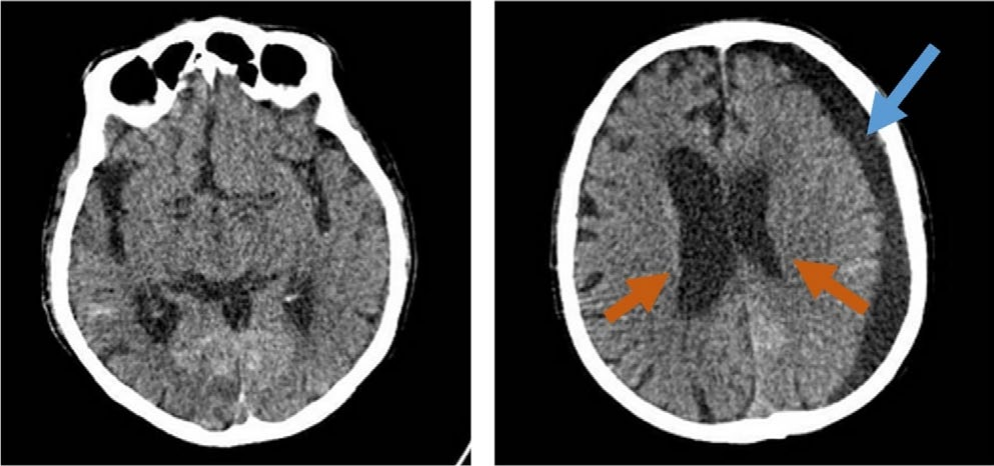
Second month postoperative Brain CT scan. Left: Basal cisterns are symmetric and open. Right: Both lateral ventricles are visible. The cerebral sulci are open, and there is a left‐side subdural hygroma.
